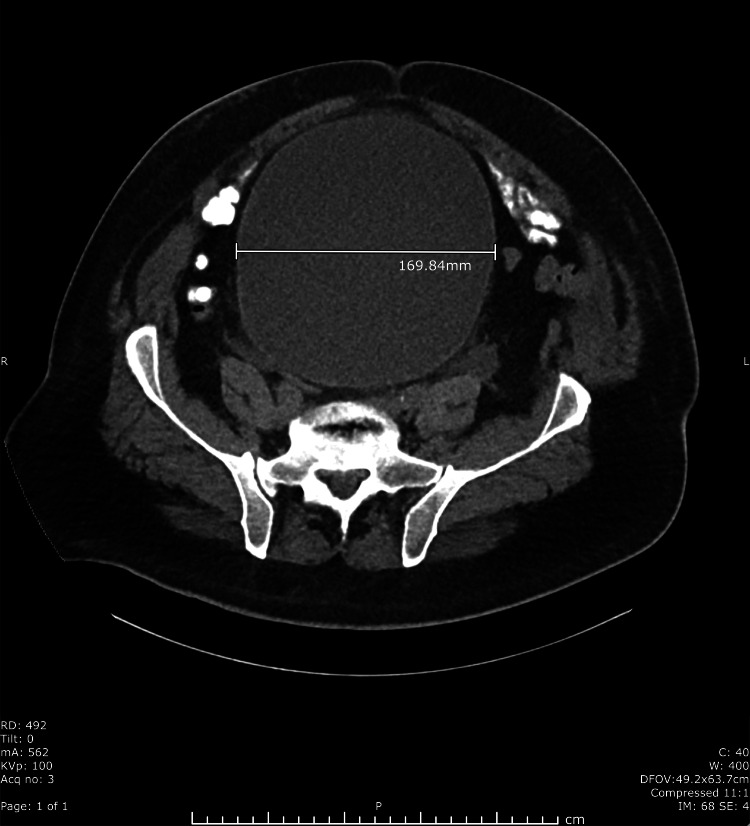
Axial view of CT abdomen and pelvis with severe urinary retention. The urinary bladder measures 169.84 mm in diameter.
